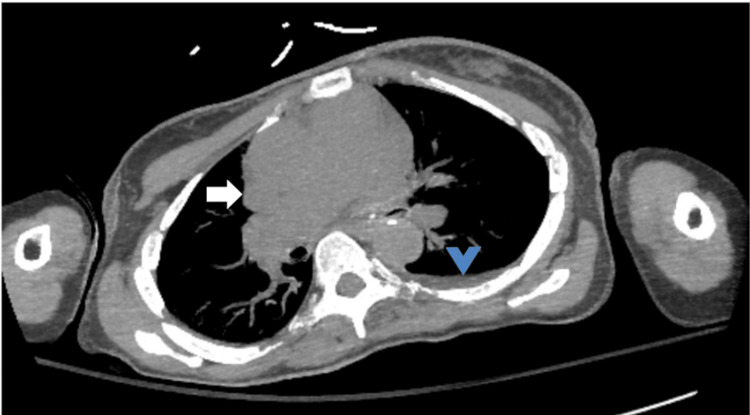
Contrast-enhanced computed tomography of the chest demonstrating cardiomegaly (white arrow) and small left pleural effusion (blue arrowhead). No focal lobar consolidation is identified.
